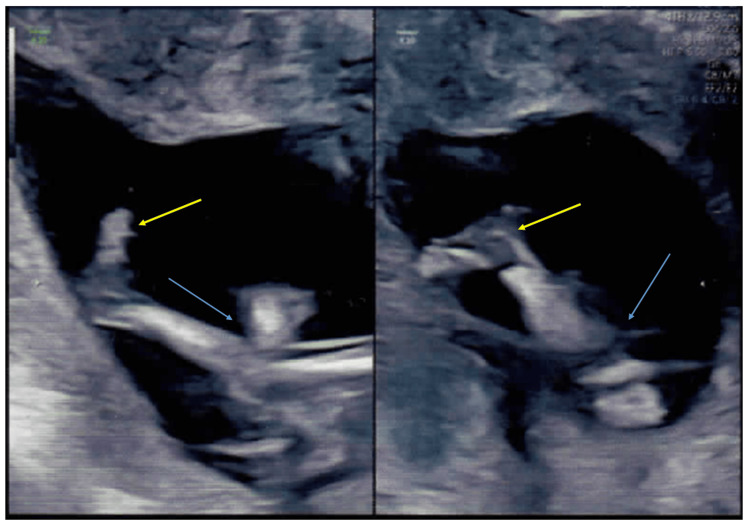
Antenatal ultrasound of the fetus in the second pregnancy around 15 weeks 3 days gestation showing hyperextended knees Antenatal ultrasound of the fetus in the second pregnancy around 15 weeks 3 days showing early signs of arthrogryposis. Both knees are hyperextended (blue arrows) and feet (yellow arrows) are normal.
